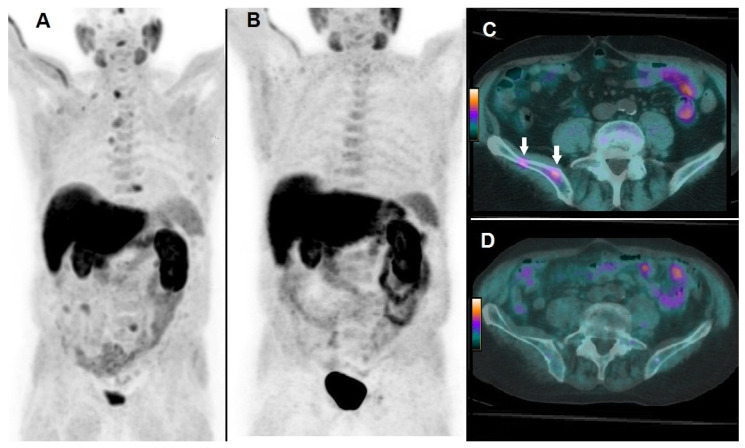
A 74-year-old patient affected by mCRPC, previously treated with androgen deprivation therapy, subsequently submitted to ^223^Ra-therapy. At baseline, ^18^F-choline PET/CT demonstrated multiple sites of tracer uptake in bones, as evident in Whole Body (A) and the following parameters were registered: PSA = 17 ng/mL; number of lesions = 18; TLA = 235.3 g. ^18^F-choline PET/CT acquired after 6 cycles of ^223^Ra-therapy demonstrated complete metabolic response as depicted by Whole Body (B). ^18^F-choline PET/CT fused axial images of the pelvis acquired at baseline showed hypermetabolic lesions in the right iliac bone (C, white arrows), while post-treatment axial slices did not show any pathological sites of tracer uptake (D). Post-treatment PSA was 3 ng/mL, OS resulted in 27 months.
